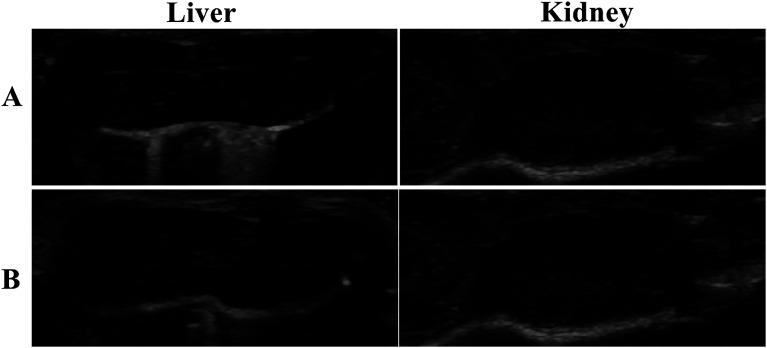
Ultrasound images of liver and kidney before (A) and after (B) injection of NCs.
